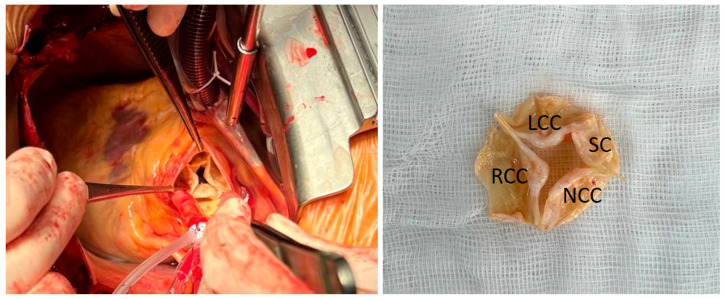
After cardiopulmonary bypass was established, the ascending aorta was cross clamped and transected. Selective cardioplegia in the left and right coronary ostia was given. During aortic root examination, the QAV was visualized and classified as type II by Nakamura classification (Figure 2). Due to partial calcification of the cusps and its malcoaptation caused by fibrous thickening, we did not attempt aortic valve repair. The aortic valve was excised (Excised aortic valve. LCC—left coronary cusp, RCC—right coronary cusp, NCC—noncoronary cusp, SC—supplementary cusp) (Figure 2) and replaced with a 23 mm biological prosthesis. Postoperative echocardiography showed no aortic regurgitation, and a mean gradient of 15 mm Hg. The patient had an uneventful postoperative course. Due to the extreme rarity of QAVs, precise preoperative assessment of the aortic valve plays a crucial role for surgical strategy. QAV is usually an isolated anomaly; however, in 18–32% of the patients it presents with a number of additional congenital heart defects including atrial and ventricular septal defects, sinus of Valsalva fistula, and subaortic fibromuscular stenosis [2]. The most frequent anomalies are coronary ostia malformations and displacement; they occur in 10% of such patients [1,3]. Awareness of these abnormalities is essential prior to the operation. Despite echocardiography being a leading modality for QAV detection, the echocardiographic findings do not always correlate with the surgical ones. Therefore, the value of the clinical features of the disease may be useful [4]. It is known that unlike patients with bicuspid aortic valve, patients with QAV are older irrespective of sex. Its most common clinical manifestation is aortic regurgitation (75%) or that combined with aortic stenosis (about 8%). Aortic stenosis alone may be present but is rare (about 1%) [2]. The vast majority of the QAV patients have a normal size ascending aorta and aortic root [5]. The surgical strategy for QAV includes aortic valve repair or replacement with biological/mechanical prosthesis, Ross procedure, Bentall procedure and even transcatheter aortic valve implantation [2,4]. Aortic valve repair is the preferable approach but the prognosis for cusp repair durability is undetermined due to lack of long-term results. At the same time, conventional valve replacement does not increase the risk of postoperative complications [5]. Taking into account the cusp morphology, we performed an aortic valve replacement due to the unpredictable outcome of the valve repair, which could jeopardize the overall result of the surgery [1]. Even though the patient did not reach the age criterion (65 years), we used the biological valve prosthesis in lieu of mechanical prosthesis to diminish the burden of antithrombotic therapy. The low incidence of the QAV emphasizes the importance of reporting such cases for a better understanding of the inherent characteristics of this rare anomaly. Evolving data will contribute to the development of QAV patient management strategies.
